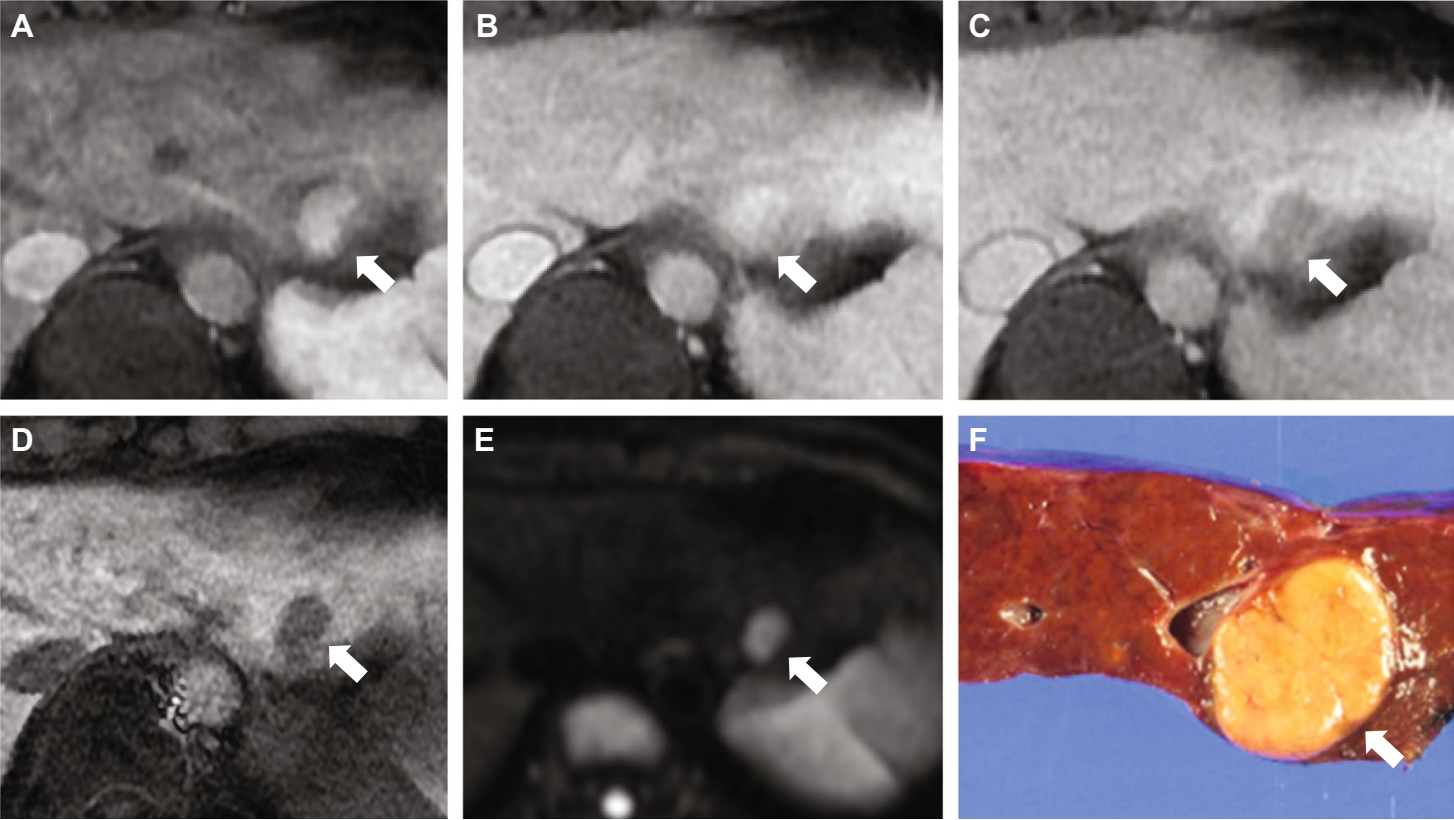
Differentiation between Eastern and Western guidelines in timing of assessment after gadoxetic acid administration. A pathology-proven HCC in a 46-year-old male patient with chronic hepatitis B. On gadoxetic acid–enhanced MRI, a nodular lesion (arrow) with non-rim APHE is seen in hepatic segment 2 (A) without washout on the PVP (B), with hypointensity on the TP (C) and HBP (D), hyperintensity on high *b*-value (*b *= 800) DWI (E). Gross pathology (F) shows a well-defined, yellow tumor, confirmed as HCC. This observation does not meet the criteria for definite HCC using LiRADS or EASL criteria, while it was diagnosed as definite HCC using Asian guidelines such as APASL or KLCA-NCC guidelines. *Abbreviations: APASL*, Asia-Pacific Association for the Study of the Liver; *APHE*, arterial phase hyperenhancement; *DWI,* diffusion-weighted imaging; *EASL,* European Association for the Study of the Liver; *HBP,* hepatobiliary phase; *HCC*, hepatocellular carcinoma*; KLCA-NCC,* Korean Liver Cancer Association-National Cancer Center Korea; *LiRADS*, Liver Imaging Reporting and Data System; *MRI*, magnetic resonance imaging; *PVP,* portal venous phase; *TP*, transitional phase. Courtesy Jeong Min Lee
